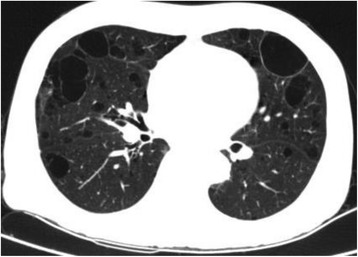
Repeat computed tomography showing multiple pulmonary cysts and bullae in both lungs, and interstitial pulmonary fibrosis
